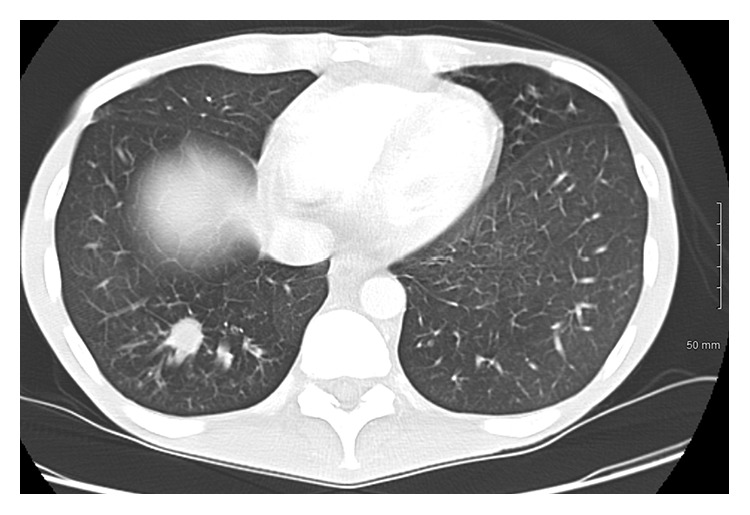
Contrasted CT of the chest showing a 16 mm right lower lobe nodule.
